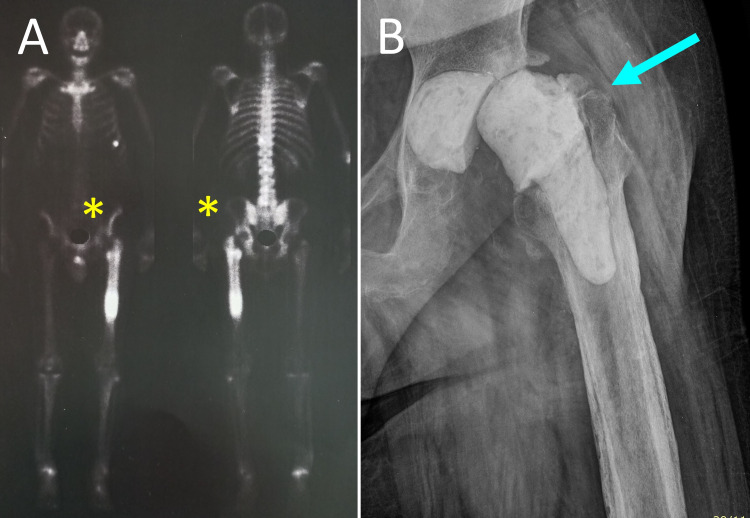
PJI of the left hip of a 65-year-old man with Staphylococcus aureus-infected left THR A. 99m Tc bone scan shows increased radioisotope uptake at the proximal metaphysis and the diaphysis of the left femur (yellow asterisks). B. Anteroposterior radiograph of the left hip after removal of the prosthesis, extensive debridement and implantation of a ball-and-socket ALCS (blue arrow). PJI: prosthetic joint infection, THR: total hip replacement, ALCS: antibiotic-loaded cement spacer
